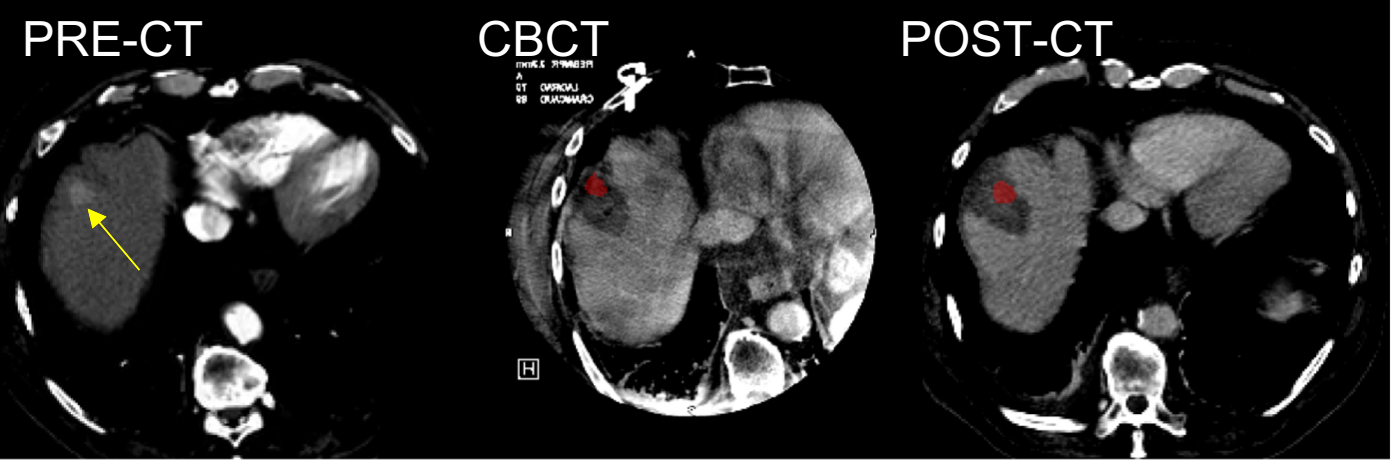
Demonstration of successful ablation treatment at CBCT. First HCC of patient #2, studied with CECT before ablation (PRE-CT) (HCC indicated by yellow arrow), intraprocedural CBCT co-registered with PRE-CT (with the pre-treatment HCC colored in red) and 24-h post-ablation with CECT (post-CT) (with the pre-treatment HCC colored in red). Both CBCT and post-CT show the ablated area entirely surrounding the target
